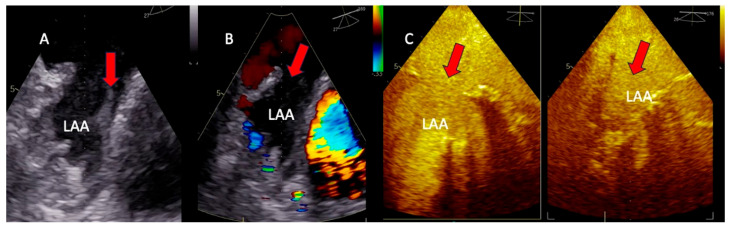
(A) TEE suspected thrombus in LAA (red arrow marking the thrombus), (B) TEE with Color Doppler showing poor function of LAA (red arrow), (C) contrast opacification of LAA for thrombus exclusion (red arrow pointing to LAA).
